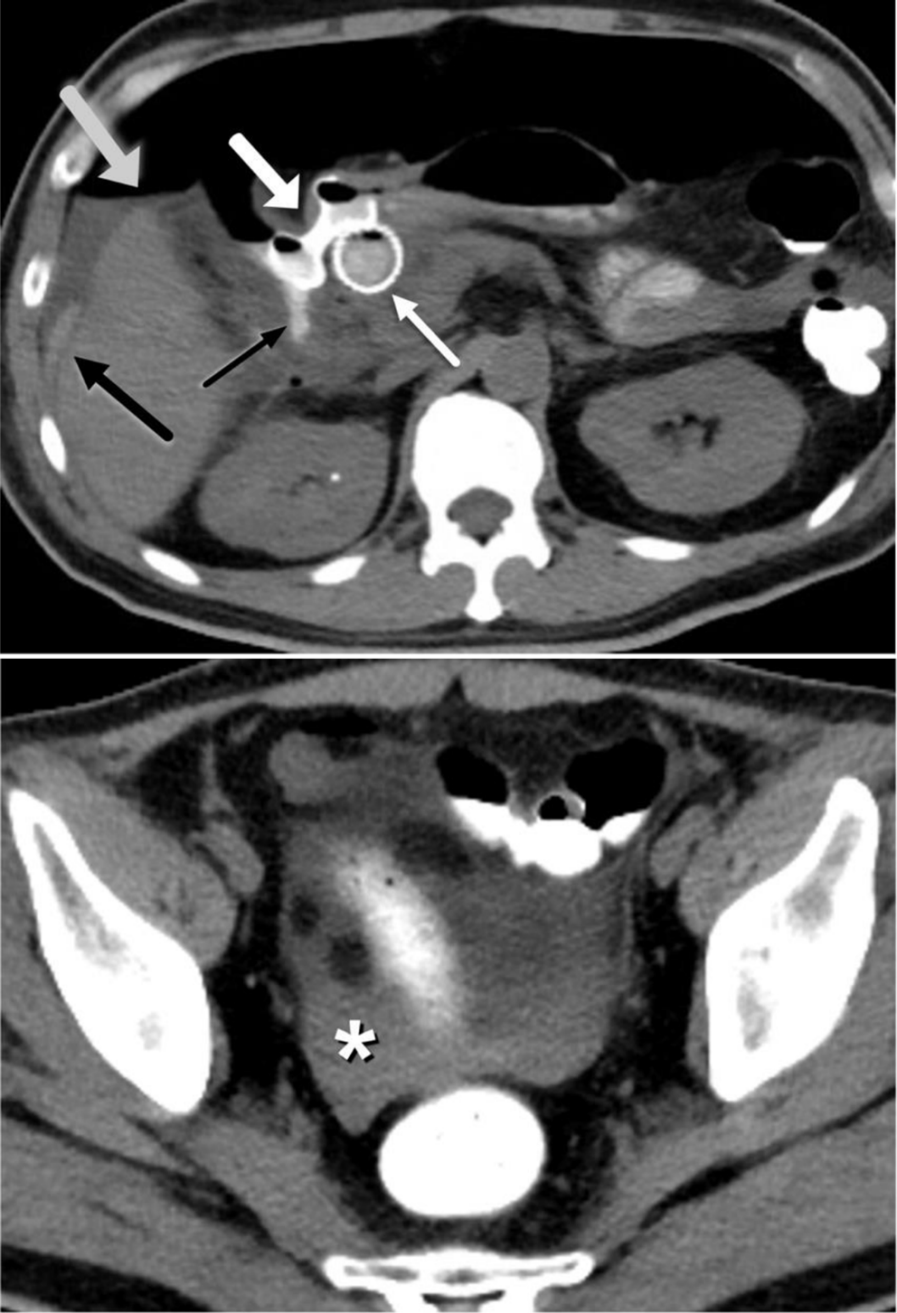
73 year-old male with pancreatic carcinoma and acute cholecystitis. A. Axial CT image demonstrates LAMS-cholecystogastrostomy (thick white arrow) performed in attempt to relieve acute cholecystitis. Axios maldeployment or dislodgement from gallbladder is evident with oral contrast (thick black arrow) noted in the perihepatic space, as well as air-fluid level (gray arrow) indicating presence of pneumoperitoneum and ascites. Extraluminal contrast leakage from the distal portion of the LAMS is noted (thin black arrow). Biliary stent containing contrast and small amount of air (thin white arrow) is noted in the CBD. B. Axial CT image shows fluid-fluid level in pelvic ascites indicating presence of extravasated oral contrast mixing with fluid (white asterisk)
